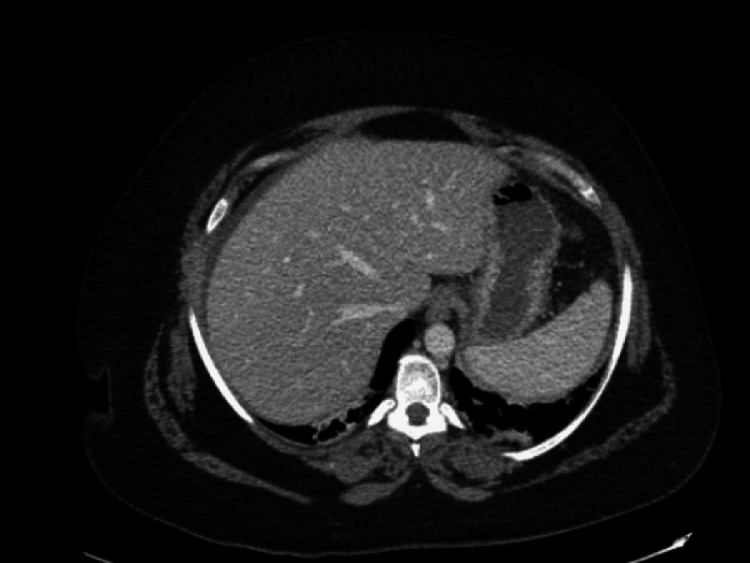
CT scan of the abdomen at admission. Note the normal liver parenchymal features. CT: computed tomography
